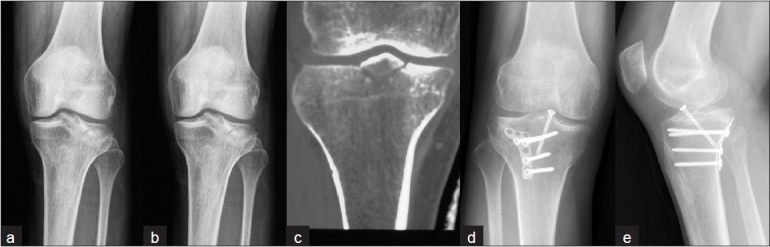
Anteroposterior (a) and lateral (b) radiographs of Patient 1 show the fracture of tibial plateau with main fragment in posterolateral, and CT scanning in coronary (c) shows avulsion fracture of the anterior cruciate ligament (ACL), which is a crucial instrument for knee stability. Postoperative anteroposterior (d) and lateral (e) views of the tibial plateau show satisfactory reduction and stabilization of the posterolateral fragments from PL approach and avulsion fracture from AM approach. PL= Posterolateral, AM = Anteromedial
